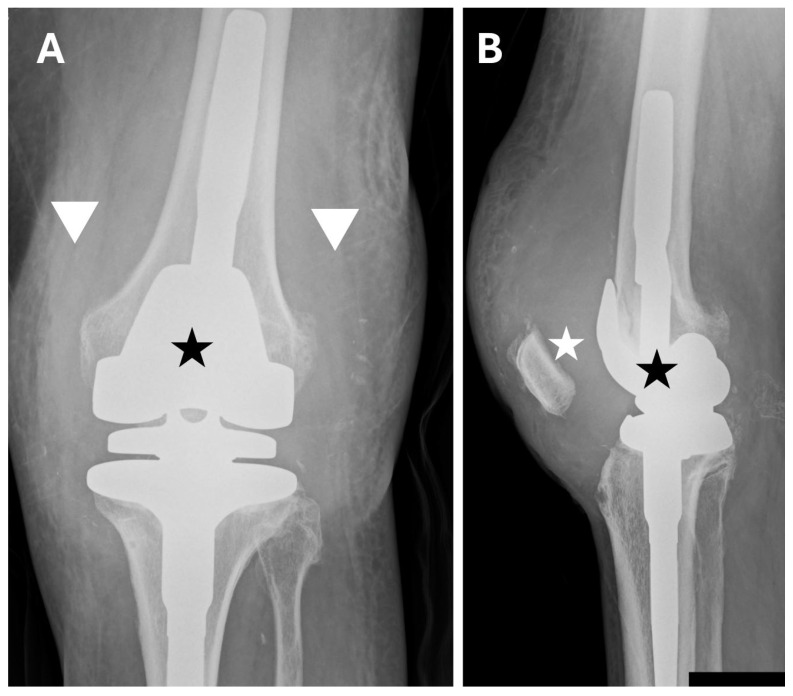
X-ray taken upon readmission after R-TKA. (A) Anteroposterior (AP) plane. (B) Lateral plane. AP and lateral views show correct alignment of the prosthetic components (black asterisks). The AP view shows significant widening of the joint outline (white arrowheads). Significant joint effusion causing an increase in the distance between the patella and the femoral component of the prosthesis (white asterisk).
